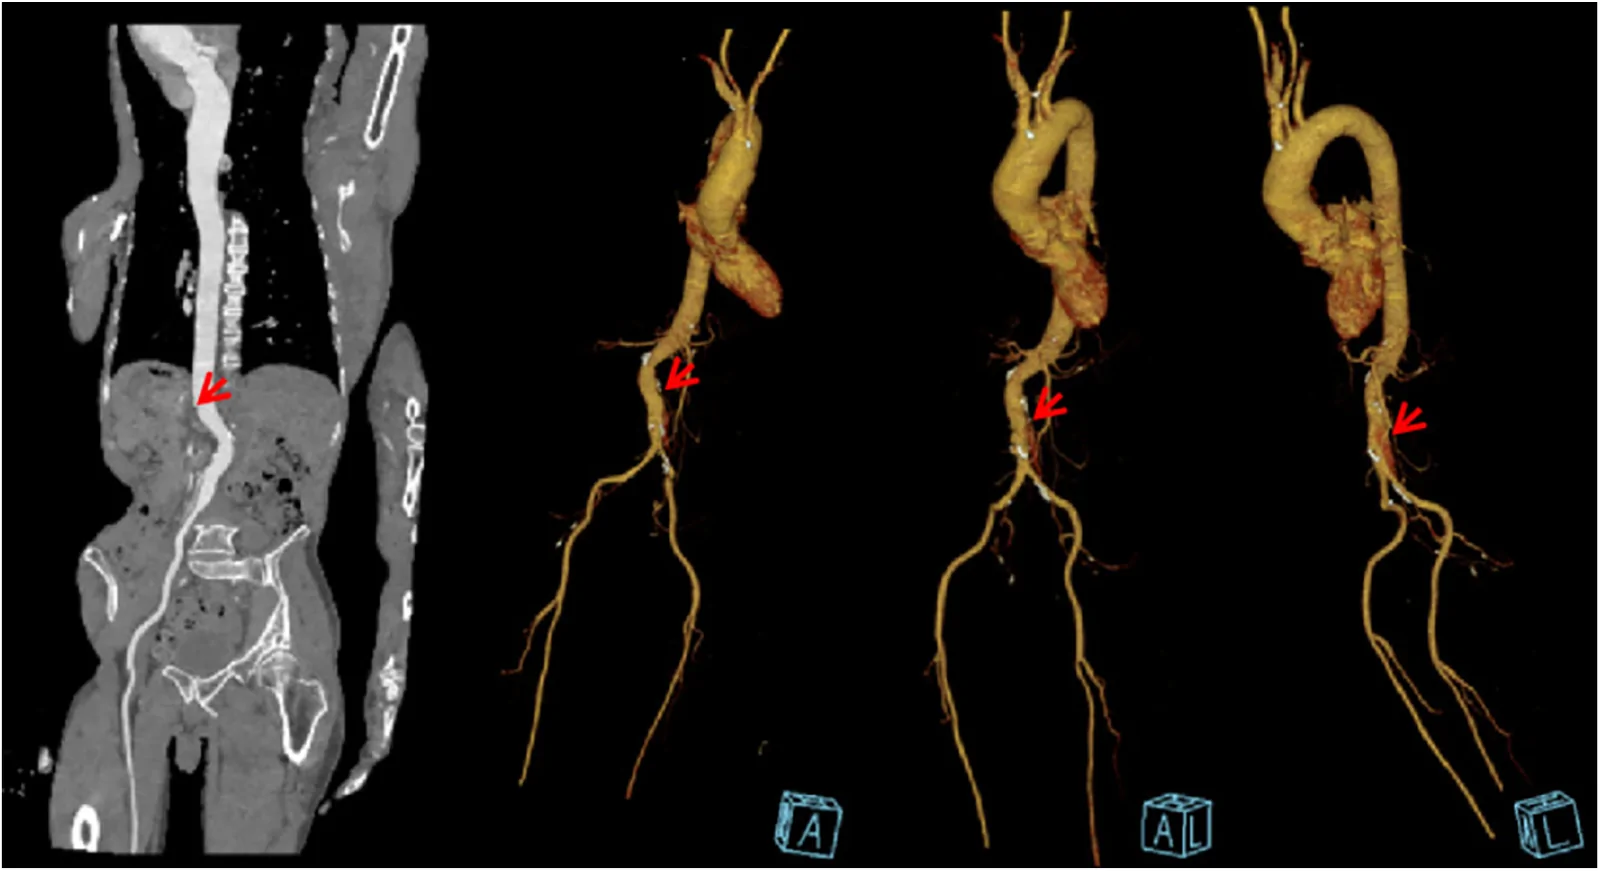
Initial admission CTA left (MPR): An intimal tear (arrow) at the abdominal aorta inferior to L3, with contrast flowing from true to false lumen. Right (3D VR):Views from theanterior, anterior-right oblique, and anterior-left oblique projections show the tear (arrows) on the left anterior wall of the distal aorta. The dissection extends distally to bilateral iliac arteries with false lumen expansion.
